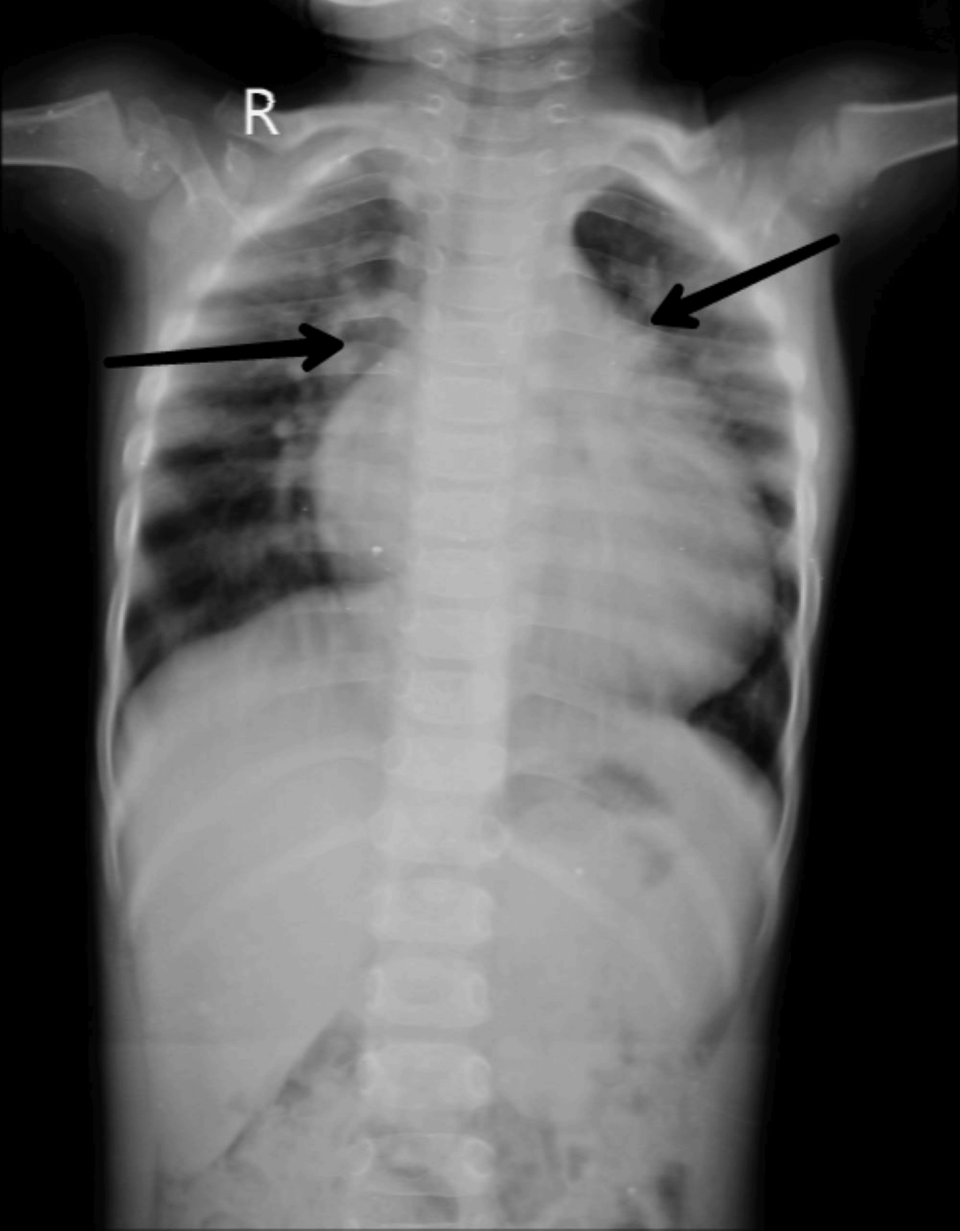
Preoperative AP chest radiograph AP: Antroposterior
